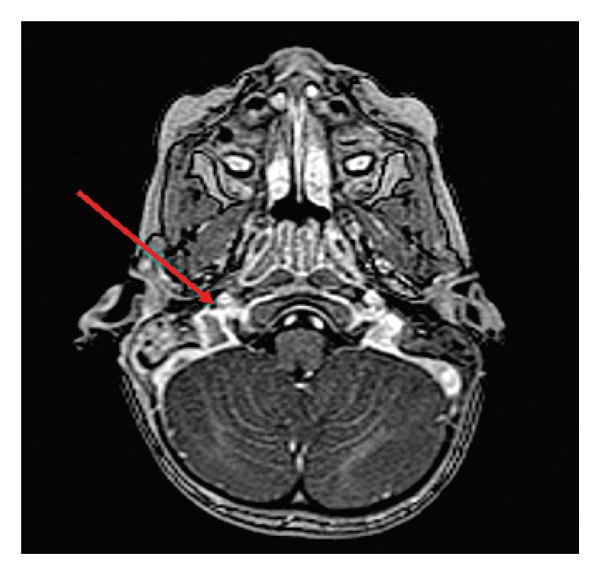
Brain MRI—internal jugular vein and venous sinus thrombosis (right‐sided).
